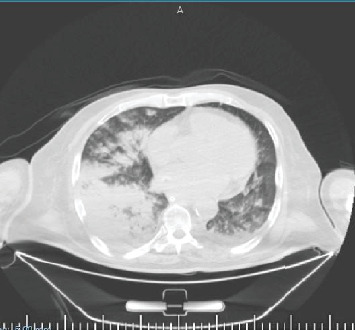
Noncontrast chest CT demonstrating consolidation in the right middle and lower lobes, consistent with pulmonary hemorrhage, and minimal bilateral pleural effusion.
